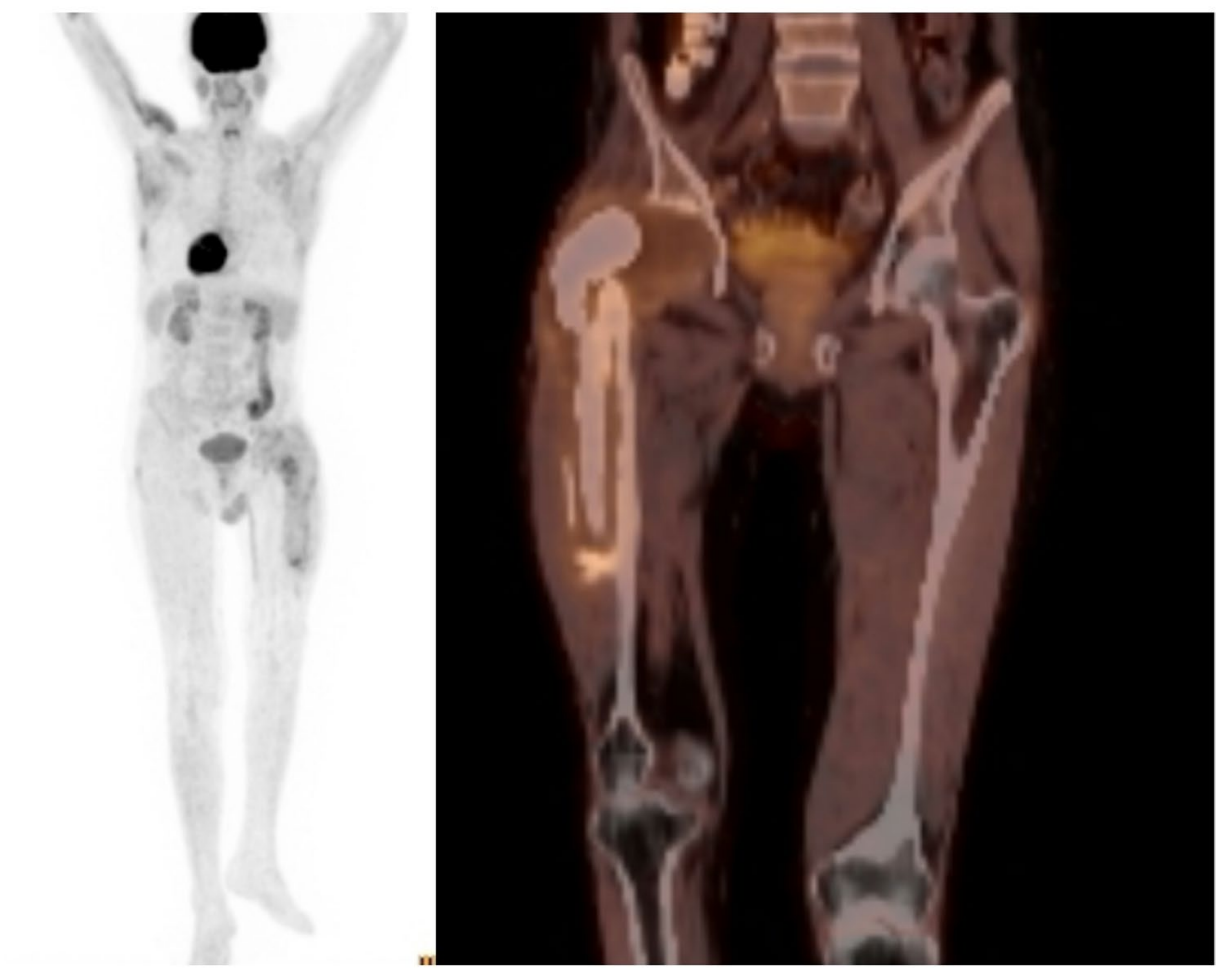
(Positive example). Axial and coronal fused 18F-FDG PET/CT image demonstrating focal increased FDG uptake at the bone–cement interface, considered suggestive of infection according to the predefined PET criteria. (SUV-max: 3.5)
